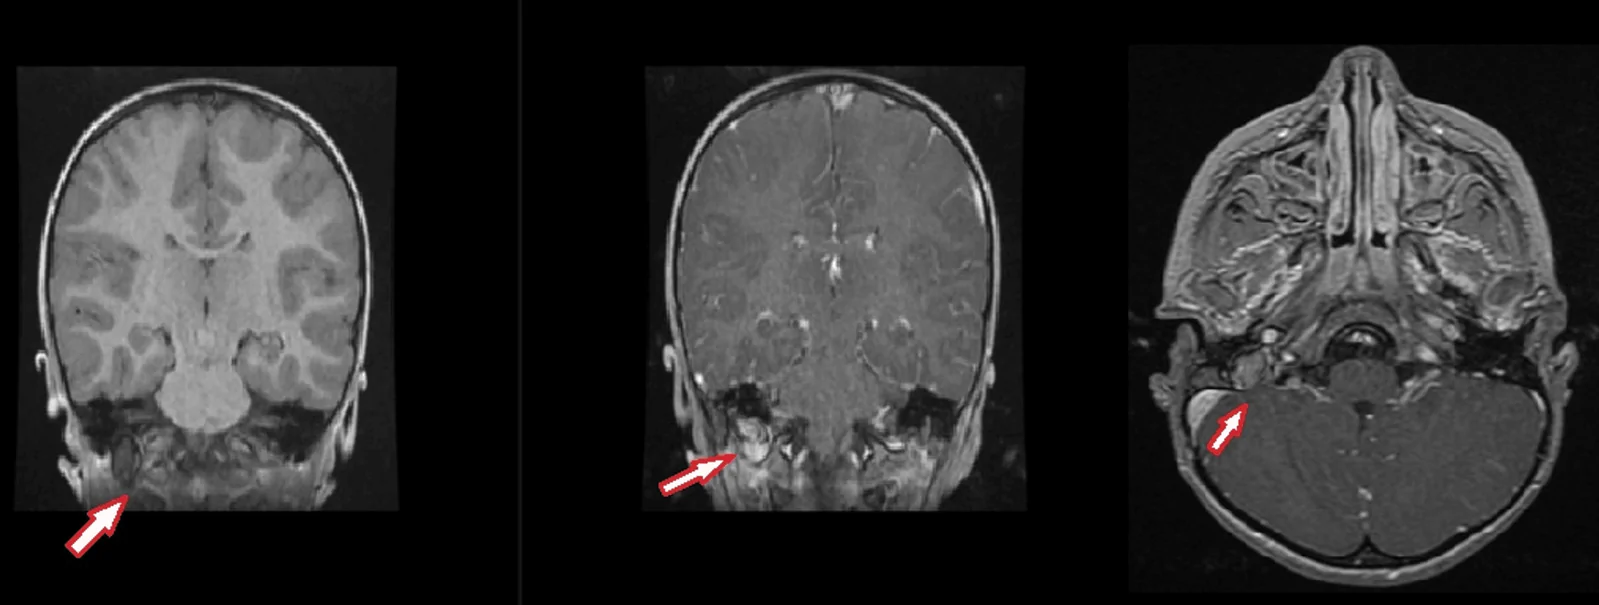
MRI of the temporal bone showing a protrusion of the jugular bulb into the right middle ear, consistent with a HRJB (arrows). Left image: Coronal section from T1-weighted MRI without contrast showing hypointense JB (indicated by the arrow). Middle image: Coronal section from T1-weighted MRI with fat saturation and IV contrast showing prominent enhancement of JB (indicated by the arrow). Right image: Axial section from T1-weighted MRI with fat saturation and IV contrast showing prominent enhancement of JB (indicated by the arrow). HRJB: high-riding jugular bulb; JB: jugular bulb.
